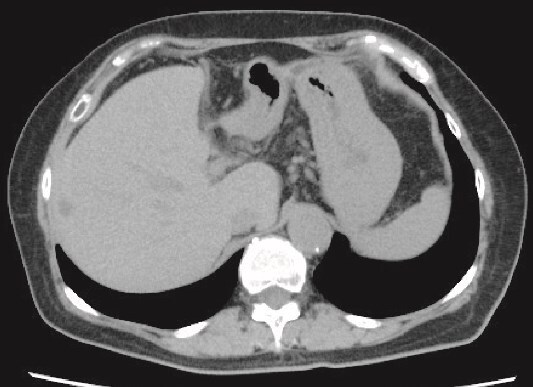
Plain CT image 6 months after the patient’s surgery. There was no evidence of hepatic cyst recurrence.
